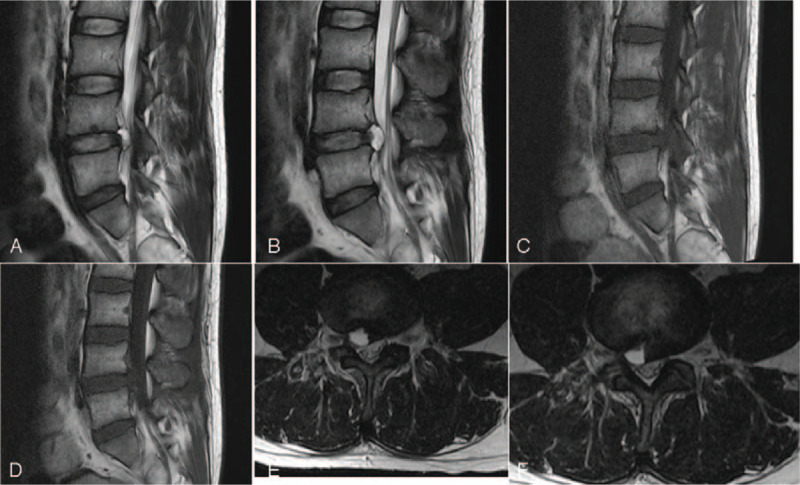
MR imaging at 1 mo after surgery. (A and B) Sagittal T2WI revealed cystic mass with high signal at L4/5 disc space; (C and D) sagittal T1WI revealed cystic mass with low signal at L4/5 disc space; (E and F) cross-sectional scanning revealed cystic mass with high signal on the right side of the central spinal canal at L4/5 disc space.
